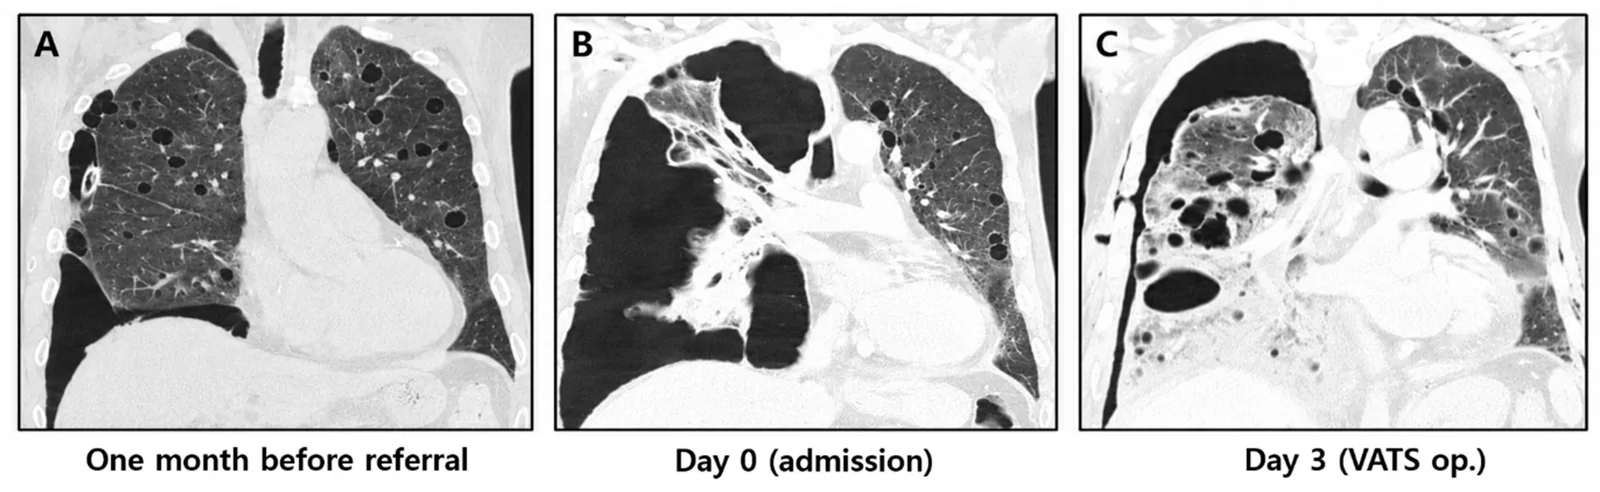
Evolution of pulmonary cystic lesions on chest computed tomography (CT): (A) Initial chest CT at the outside hospital demonstrating multiple bilateral thin-walled cystic lung lesions with a right-sided pneumothorax. (B) Admission CT showing recurrent right-sided pneumothorax with progression of the diffuse cystic lesions. (C) Follow-up CT demonstrating rapid progression of extensive cystic and hemorrhagic pulmonary lesions before thoracoscopic biopsy confirmed pulmonary metastatic angiosarcoma.
